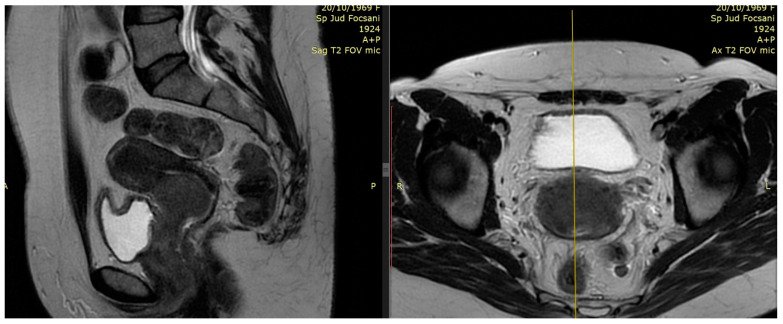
Patient 2 with FIGO stage IVA was treated with EBRT and 3 fractions of HDR CT-based brachytherapy. T2-weighted pretreatment MRI: 4.5 × 5 × 4.6 cm tumor indicating complete vaginal invasion up to urethral meatus and posterior bladder wall involvement—sagittal view and axial view.
